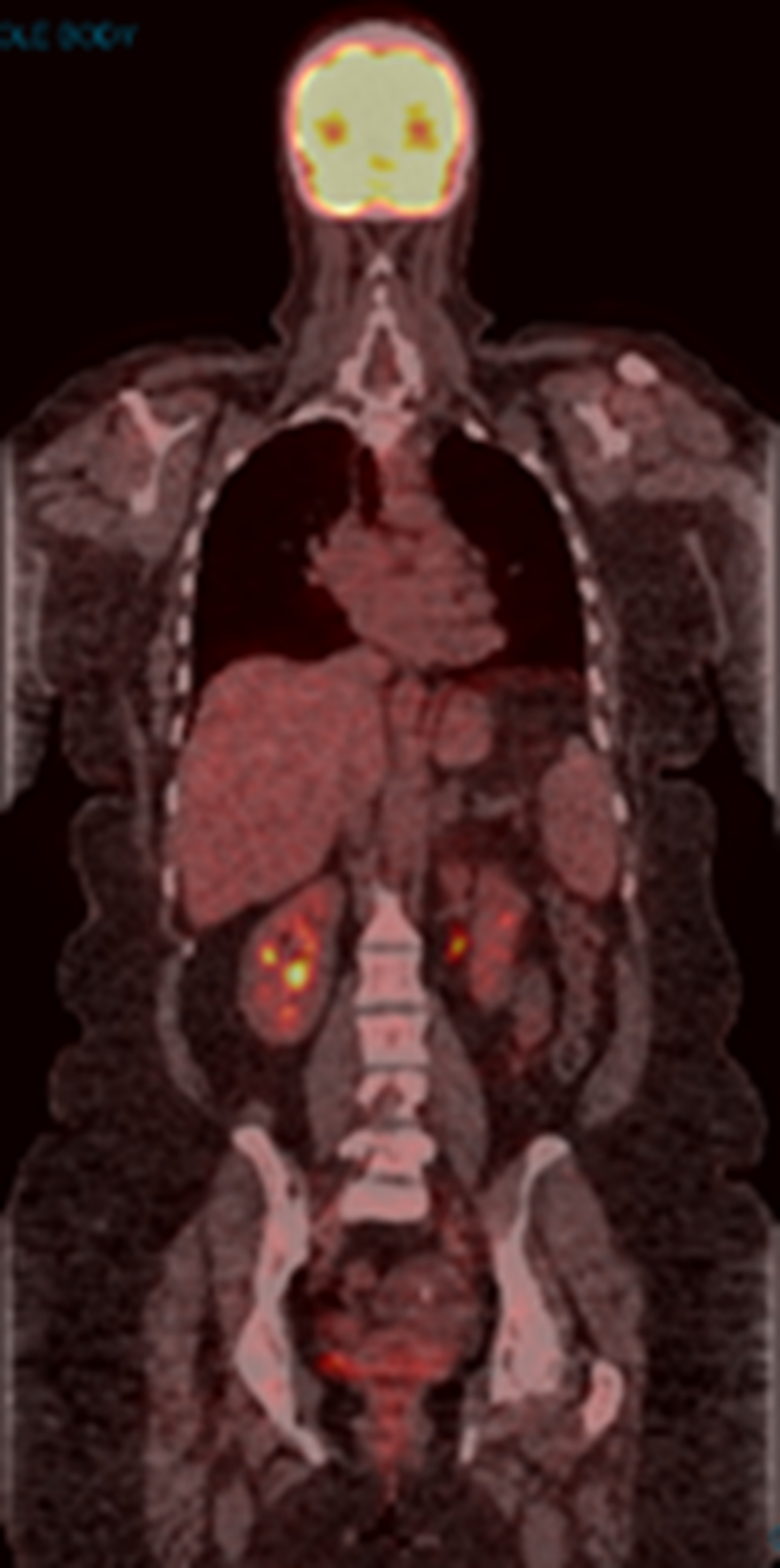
PET/CT scan in May 2017 The image shows the complete resolution of all FDG-avid lymphadenopathy PET: positron emission tomography; CT: computed tomography; FDG: fluorodeoxyglucose
